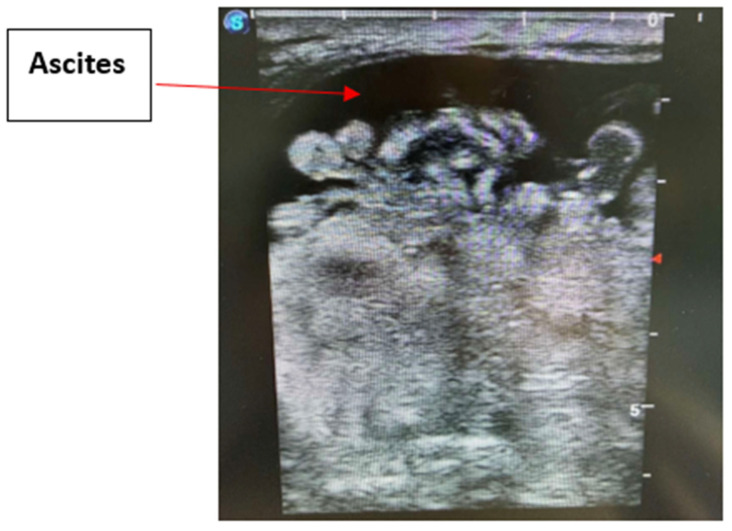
Abdominal ultrasound with free fluid—ascites.
